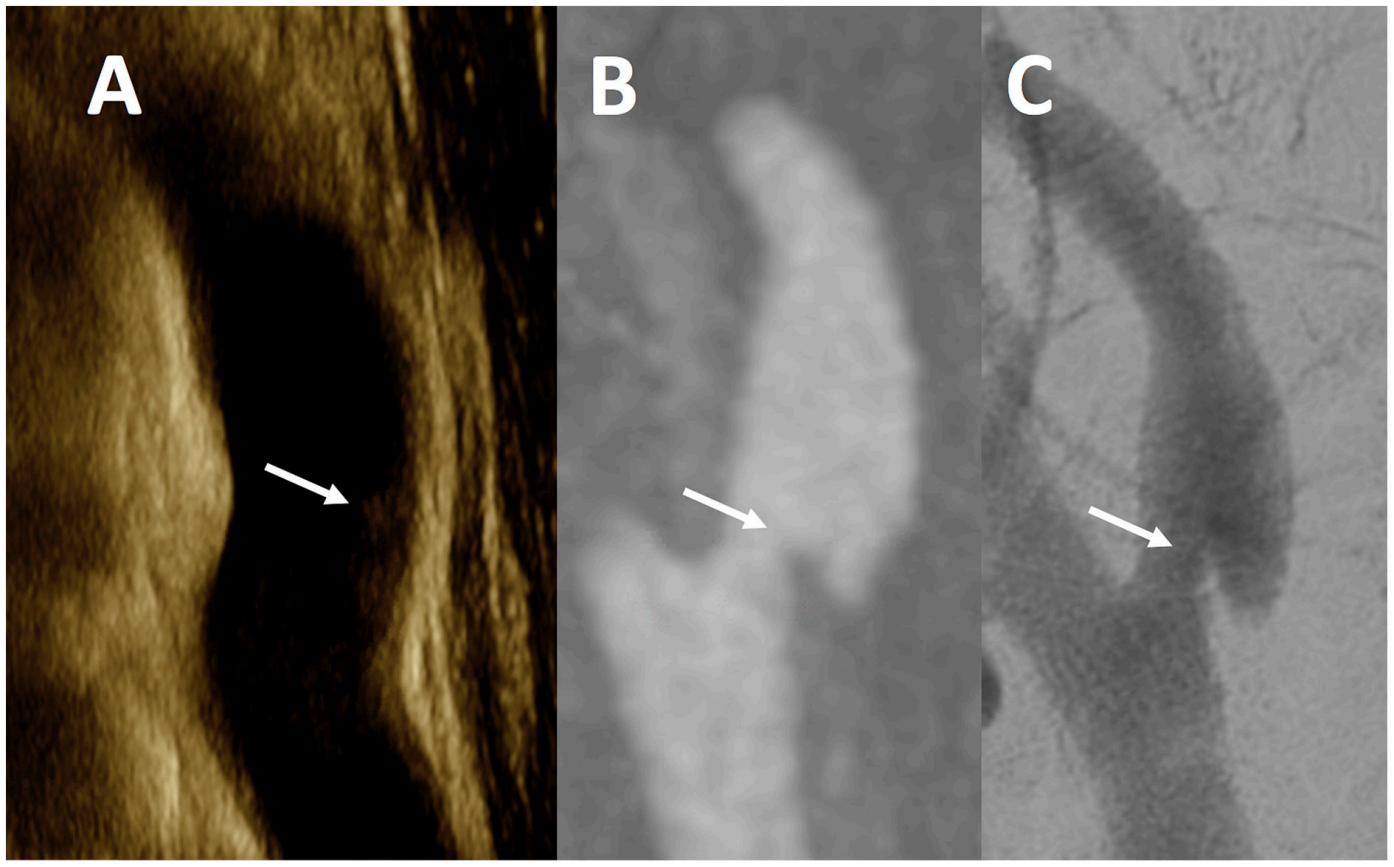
Multimodality Imaging of Carotid Web. This figure is a representative example of a CaW across all modalities in a single patient. The white arrows noted on ultrasound (A) as an subtle echogenic focus along the posterior wall of the internal carotid artery, and a shelf-like defect in the posterior wall of a sagittal maximum intensity projection image from CTA (B), and single lateral view from DSA (C) of the same internal carotid artery.
